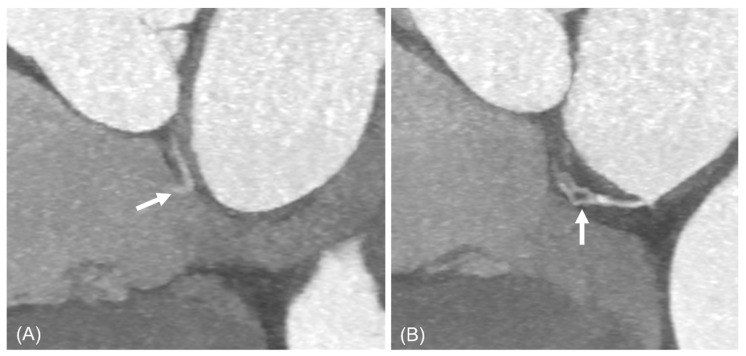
An 87-year-old woman with network-like-type diverticulum communicating with RA. The lesion was identified during the preoperative work-up for aortic arch aneurysm surgery. Short-axis images of cardiac CT scan show a shunt (A) (arrow) and spiderweb-shaped channels (B) (arrow) from inferior LA toward RA. Abbreviations: LA, left atrium; RA, right atrium.
